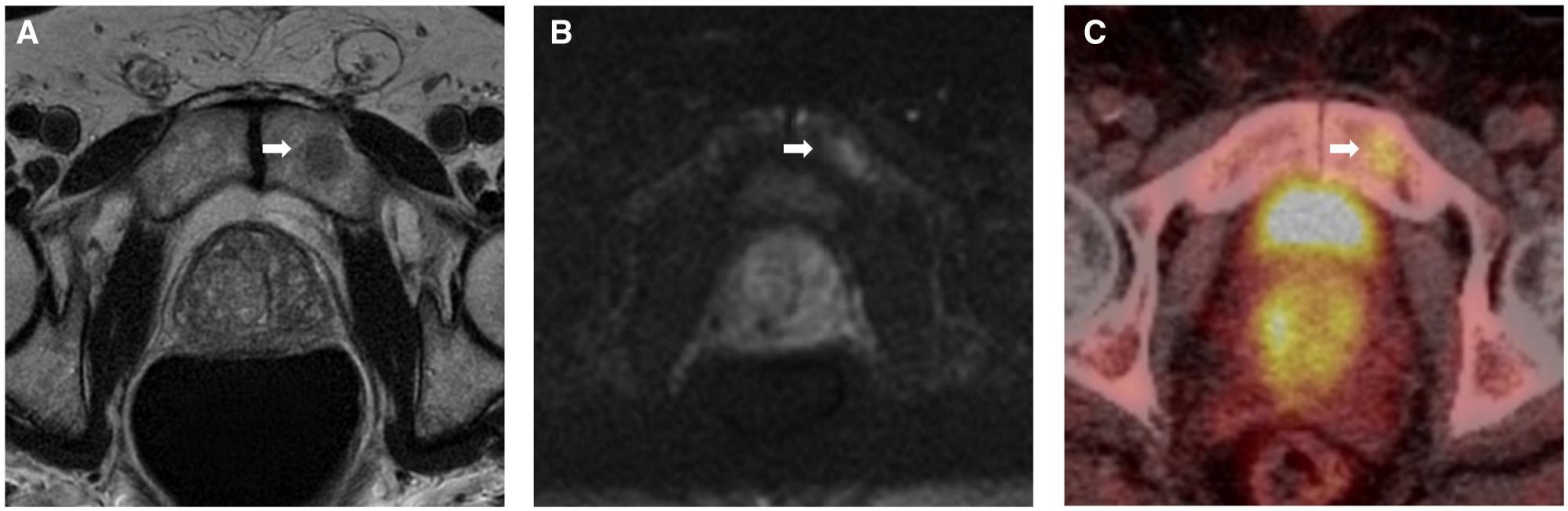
Left pubic body lesion (white arrow) identified within a 75-year-old male undergoing investigation for raised PSA. (A, B) Axial MRI sequences demonstrate intermediate reduced T1w signal (A) and increased B1000 signal (B). (C) Choline PET imaging performed 6 months later identifies an avid lesion with SUV of 5 (C).
